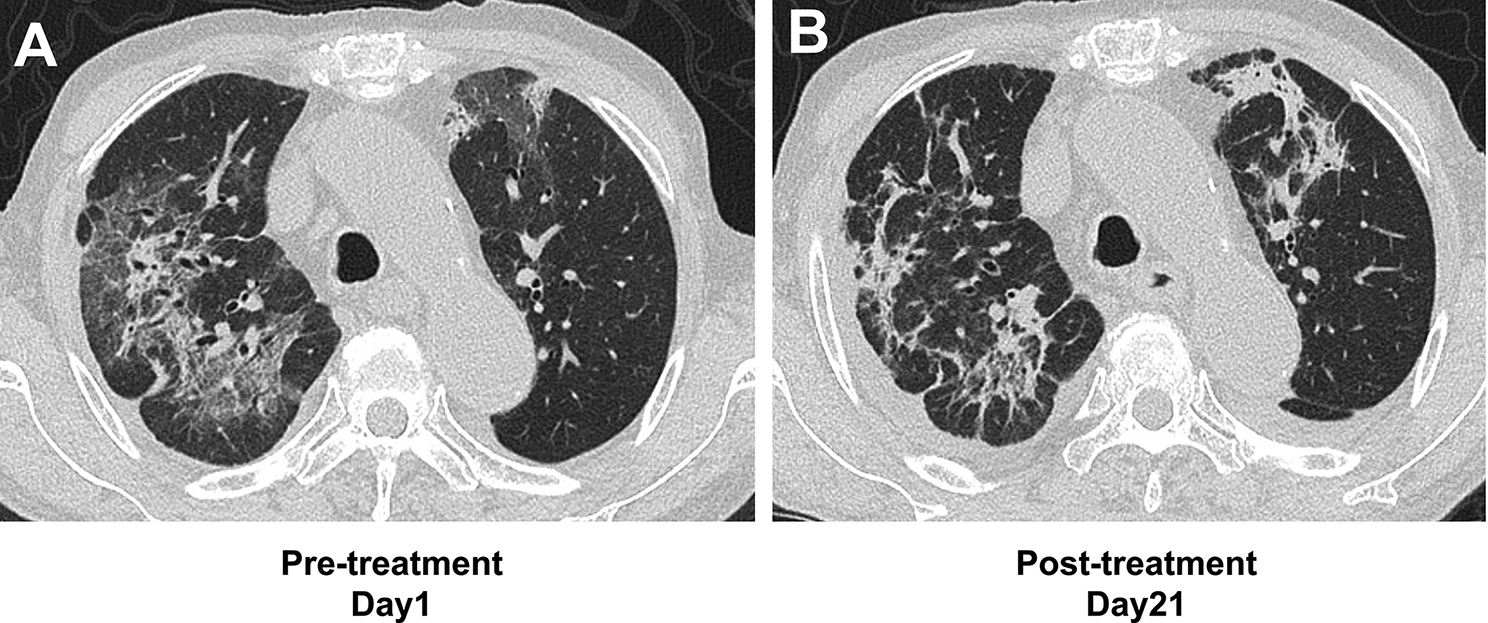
Chest computed tomography (CT) findings before and after treatment. (A) Pre-treatment (day 1): CT shows bilateral ground-glass opacities. (B) Post-treatment (day 21): following treatment with sulfamethoxazole-trimethoprim and pentamidine, the opacities have improved, with residual post-inflammatory changes
